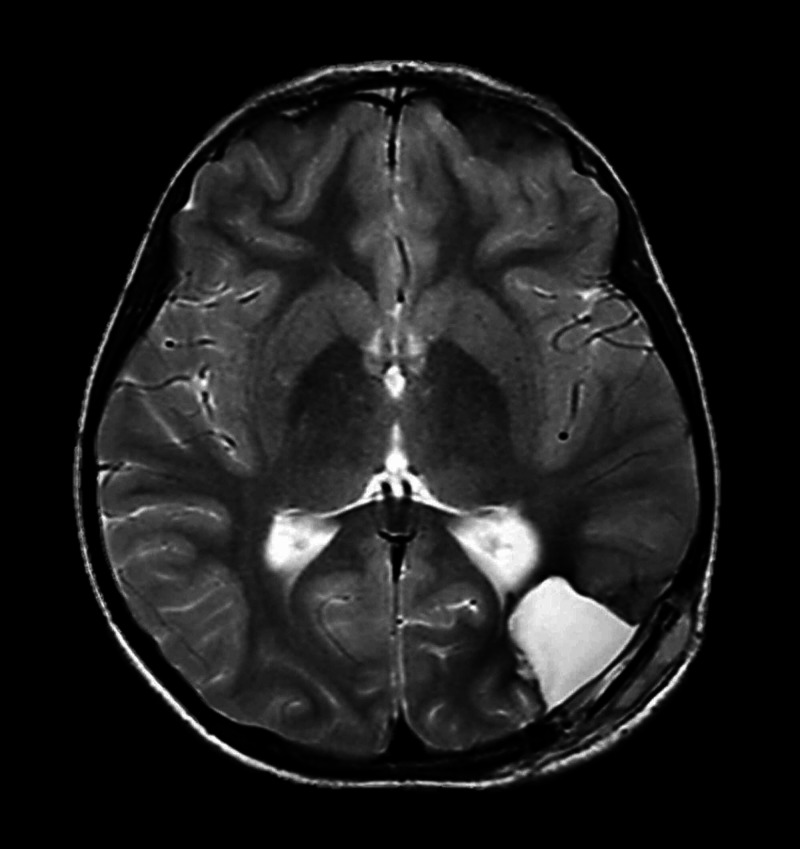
Postoperative axial T2-weighted MRI. Postoperative axial T2-weighted MRI demonstrates evacuation of hematoma and removal of the causative cavernous malformation.
